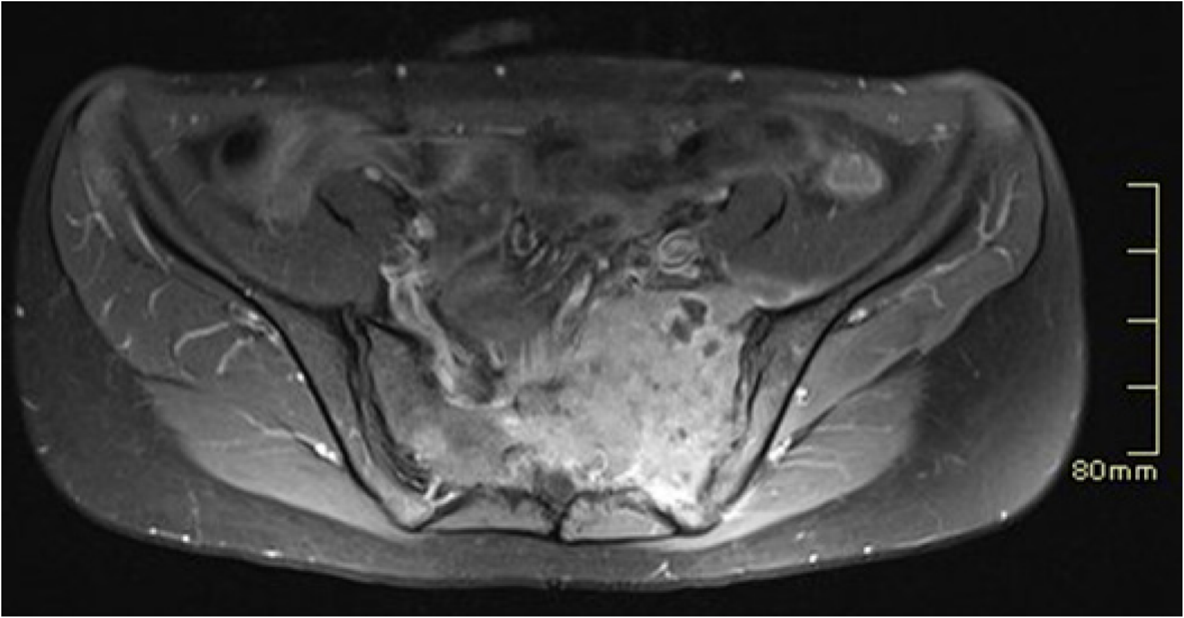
Pelvic magnetic resonance imaging. Infiltrative mass with areas of cystic appearance in the left sacral bone, extending to the sacroiliac joint and left iliac bone, obliterating the left neural foramina. Measures 6.2 × 7.9 × 5.3 cm
